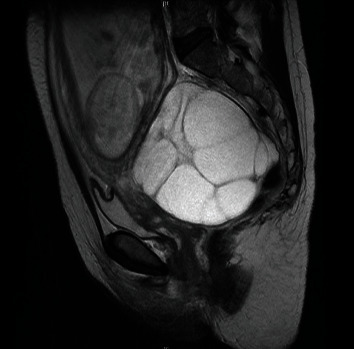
T2-weighted images show bilateral ovarian enlargement, which looks like HL. MRI of the patient's pelvic at the gestational age of 19 weeks.
